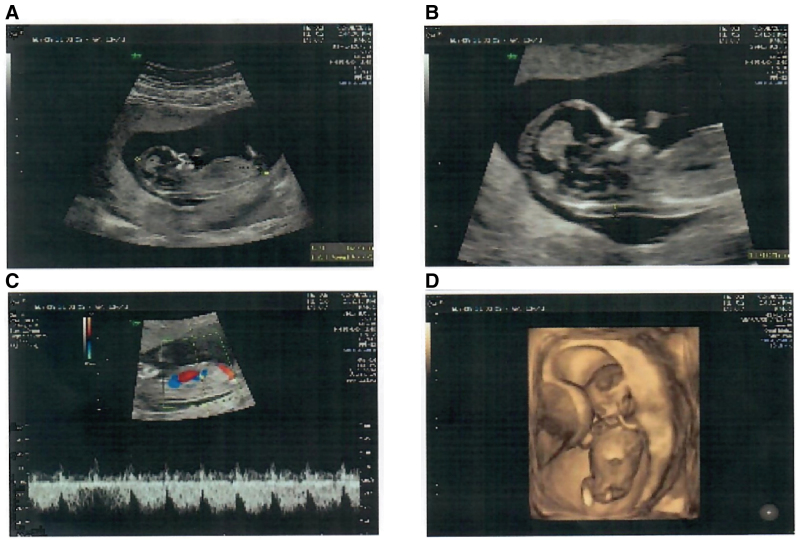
Sonographic findings of a confirmed intrauterine pregnancy. (A, B) Grayscale ultrasound images demonstrate an intrauterine fetus with a crown-rump length (CRL) of 64 mm, a nuchal translucency (NT) of 2.1 mm (within normal limits), and a visible nasal bone. Major anatomical structures, including the skull, midbrain, 4-chamber heart view, stomach, and all 4 limbs, are identified. The placenta is posterior, grade 0, with a thickness of 16 mm. Amniotic fluid distribution is normal. (C, D) Color Doppler (C) and 4-dimensional (D) images confirm fetal cardiac activity with a heart rate of 167 beats/min. The umbilical artery peak systolic velocity is approximately 40.7 cm/s, and venous duct flow shows no significant abnormality. CRL = crown-rump length, NT = nuchal translucency.
